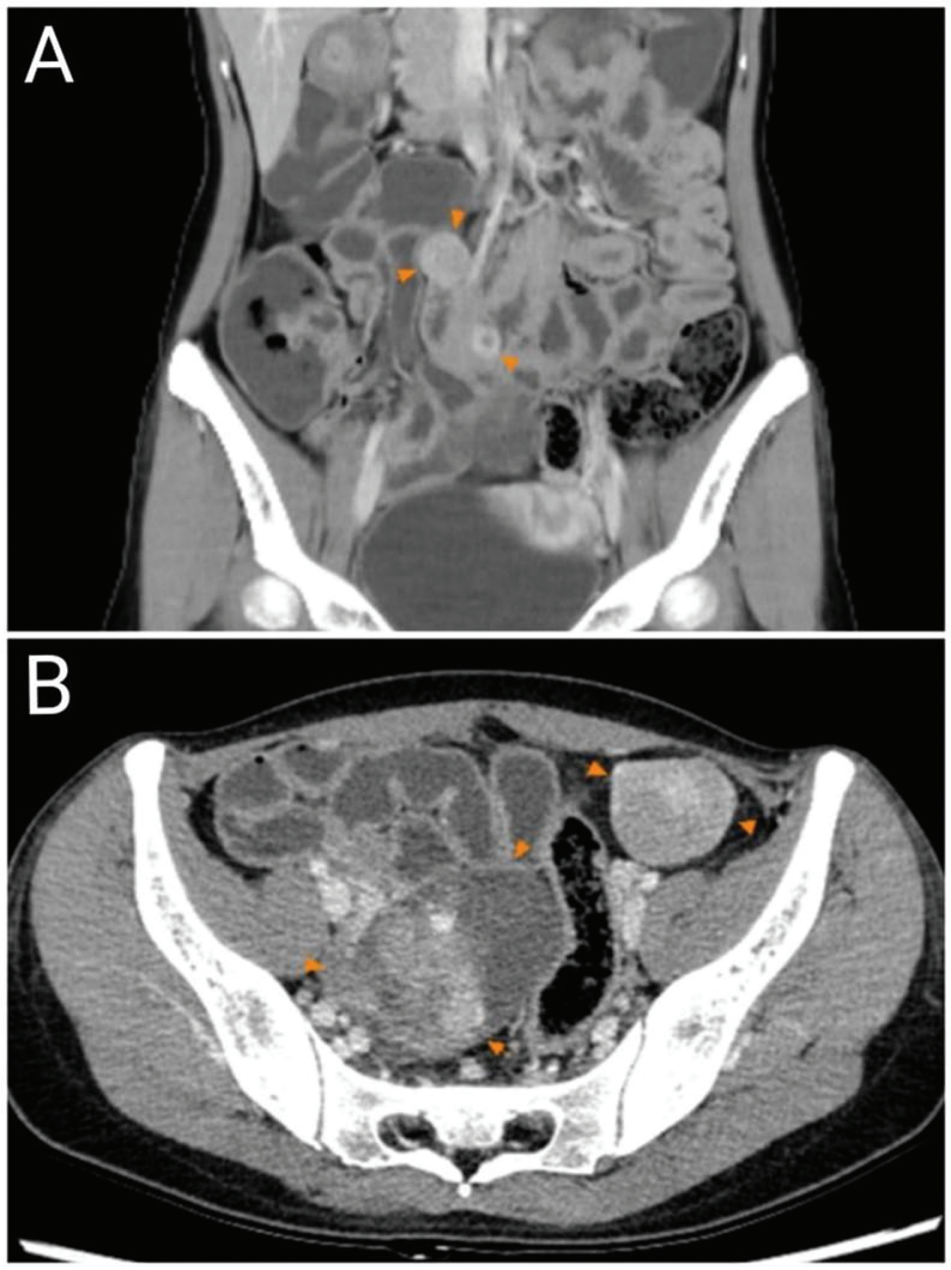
The imaging results of contrast-enhanced CT of the abdomen: (A) The arrows point to 2 tumors in the lower abdomen with diameters of 19 and 16 mm. (B) The arrows point to a mass of approximately 66 mm × 49 mm × 54 mm on the right side of the uterus and a mass of approximately 37 mm × 32 mm next to the sigmoid colon.
